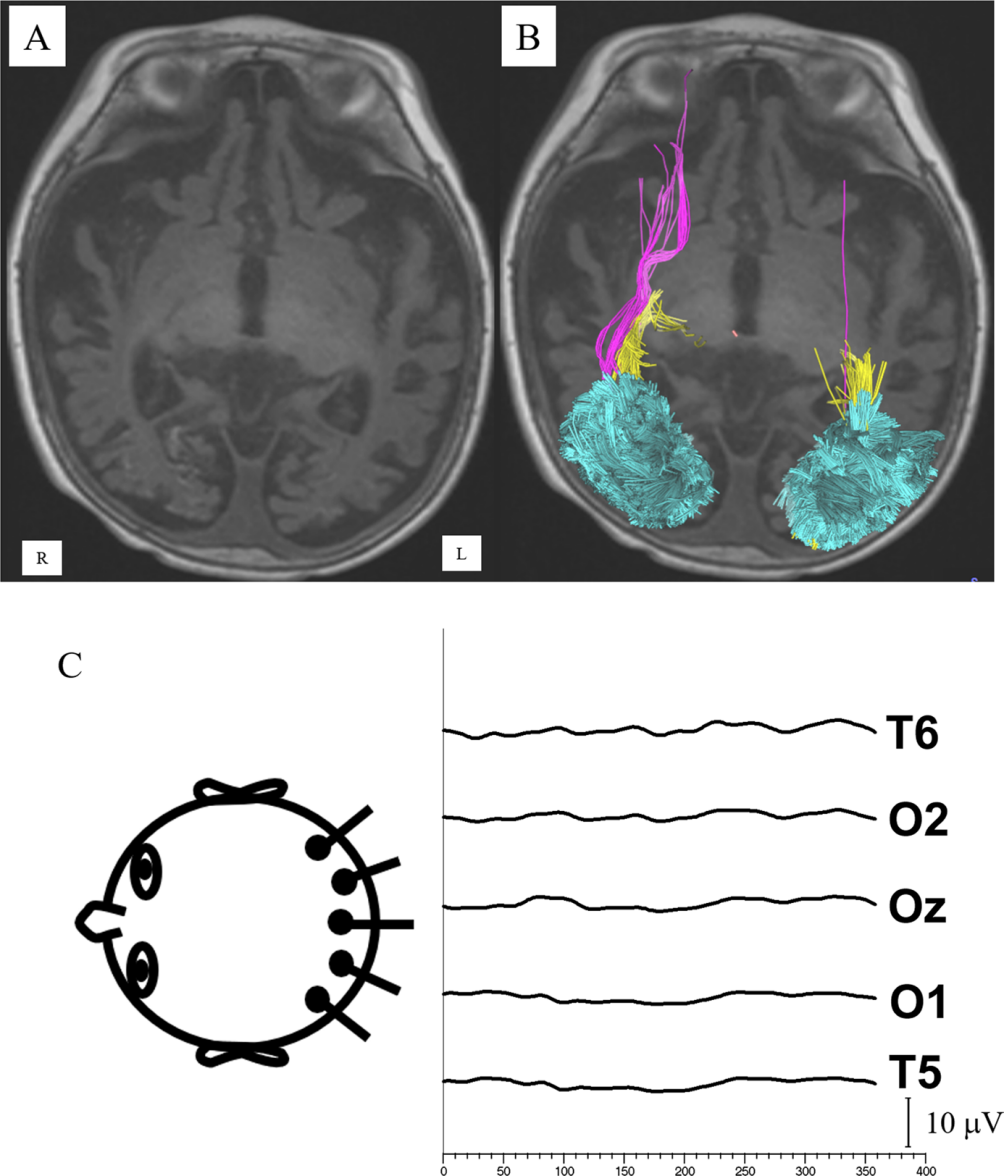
A child presenting at 108 days age with unresponsiveness, respiratory distress, subarachnoid hemorrhage, rib fractures and abdominal injury, but normal appearing fundus in both eyes. A VEP at 0.5 years age showed responses near background noise levels. MRI at 1 year of age showed significant cerebral volume loss, laminar necrosis within the frontal, parietal, and occipital lobes. Diffusion tensor imaging showed reduced volume of the optic radiations (yellow), reduced volume of the inferior fronto-occipital fasciculus (purple), and mild volume loss of the vertical occipital fasciculus. (cyan). At last follow-up, visual acuity was 1.53 logMAR and he had global developmental delay, intractable epilepsy, and spastic quadriparesis. A Axial T1-weighted image, B same image with reconstructed tracts superimposed, C VEP responses across the occiput
